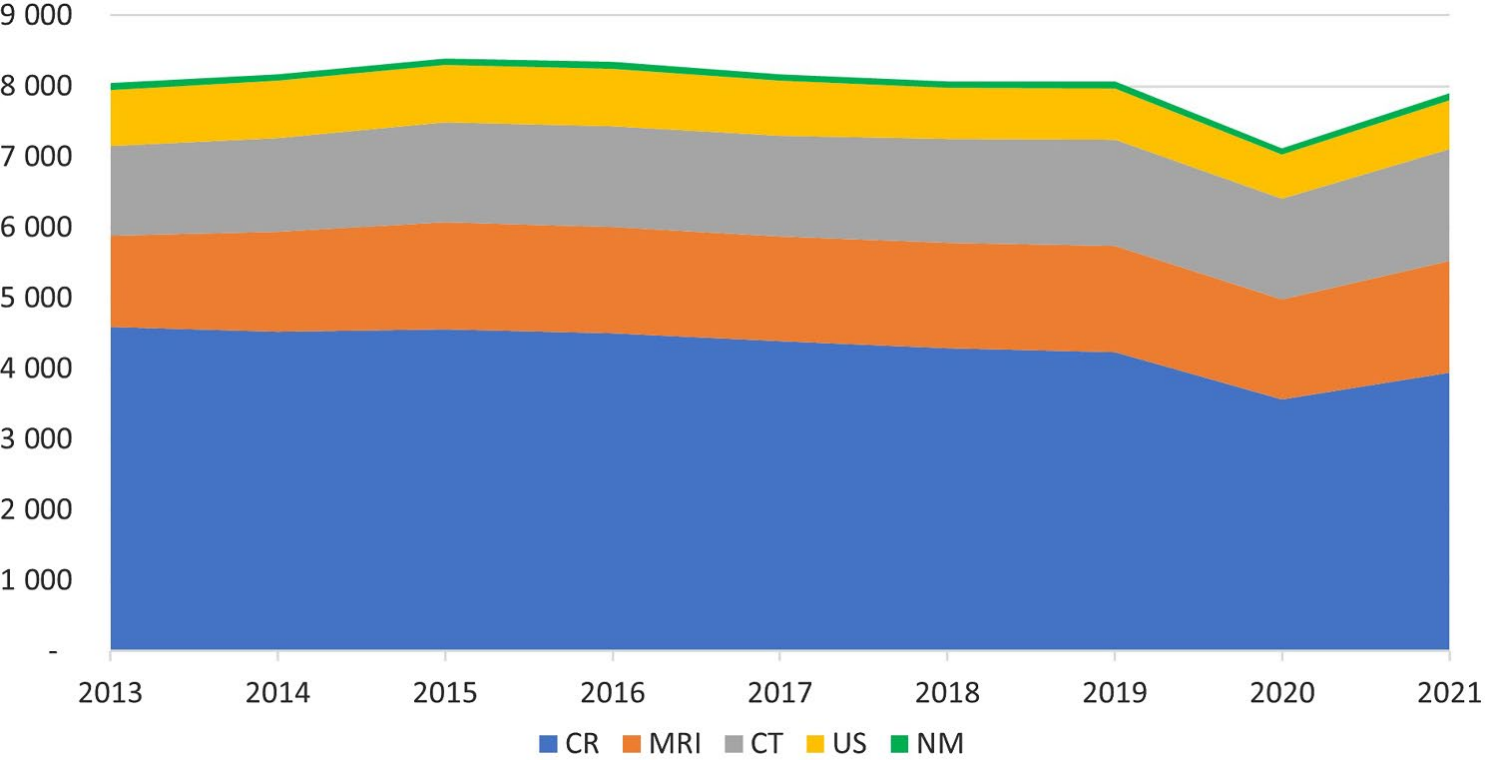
Temporal variations in imaging for the years 2013–2021 per 10,000 inhabitants for main modalities, conventional radiology including fluoroscopy (CR), magnetic resonance imaging (MRI), computed tomography (CT), ultrasound (US), and nuclear medicine (NM)
